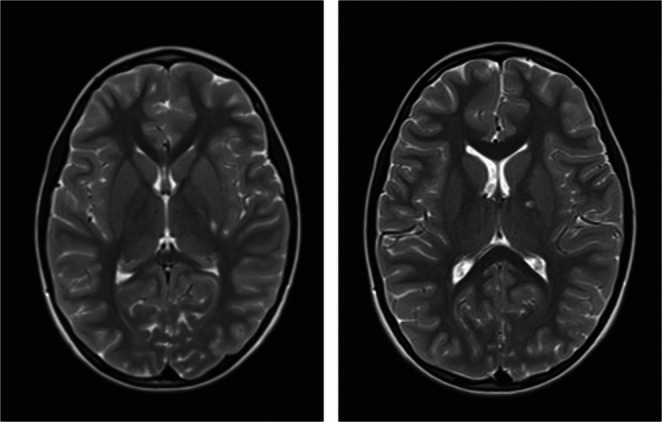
Brain MRI image. Diffuse vascular changes in the left hemisphere, namely in the left putamen and posterior limb of the left internal capsule
